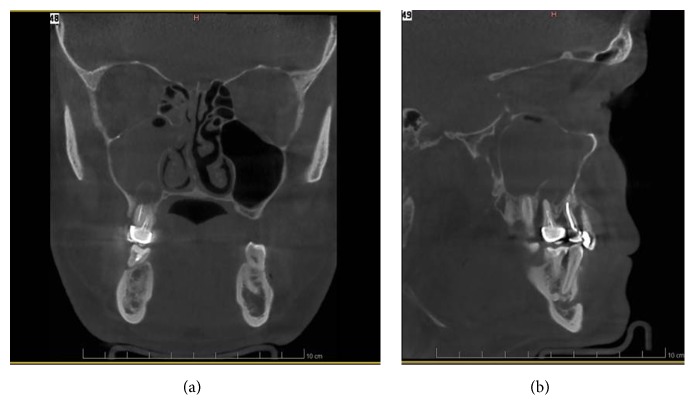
Coronal (a) and sagittal (b) CBCT-scan: well-defined hypodense lesion involving a distal and apical region (endo-perio lesion) of the endodontically treated right maxillary first molar. Maxillary and ethmoidal sinusitis and a perforation of the lesion into the right maxillary sinus cavity are also observed.
